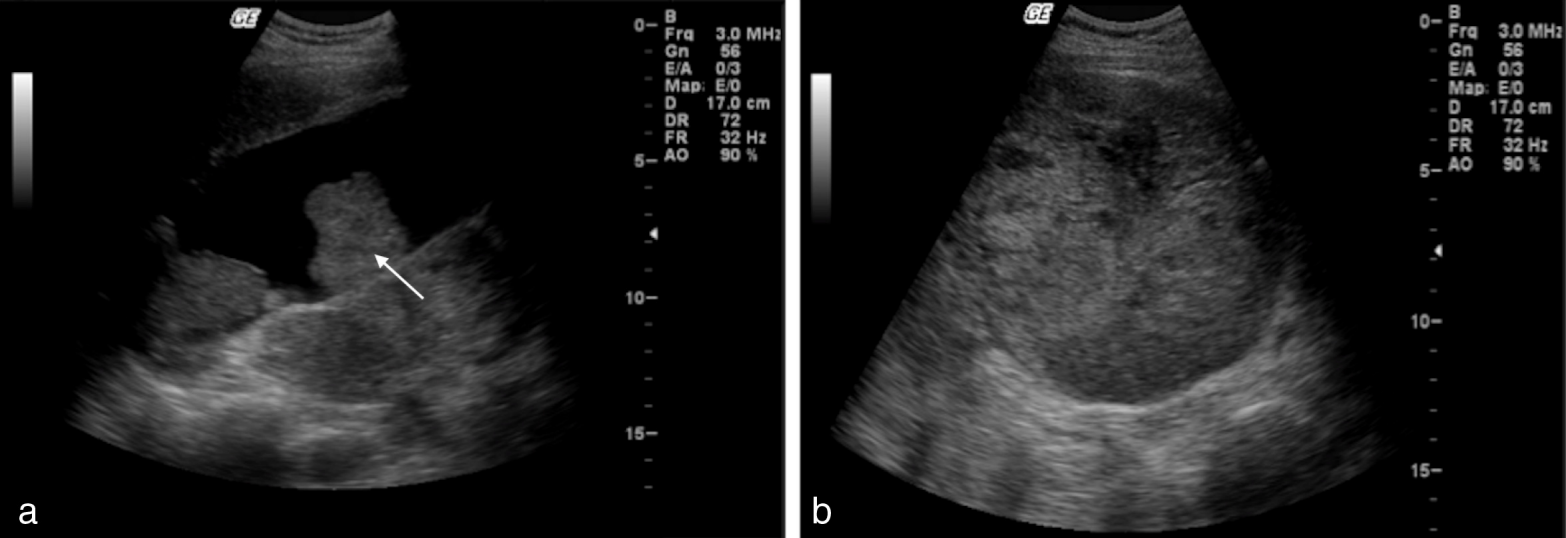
Abdominal ultrasonography images obtained at admission using a 5-3 MHz curvilinear probe show a distended gallbladder (a) with diffusely thickened wall and intraluminal polypoid lesions (arrow, a) in continuity with an exophytic, expansile, heterogeneous predominantly solid lesion (b).
